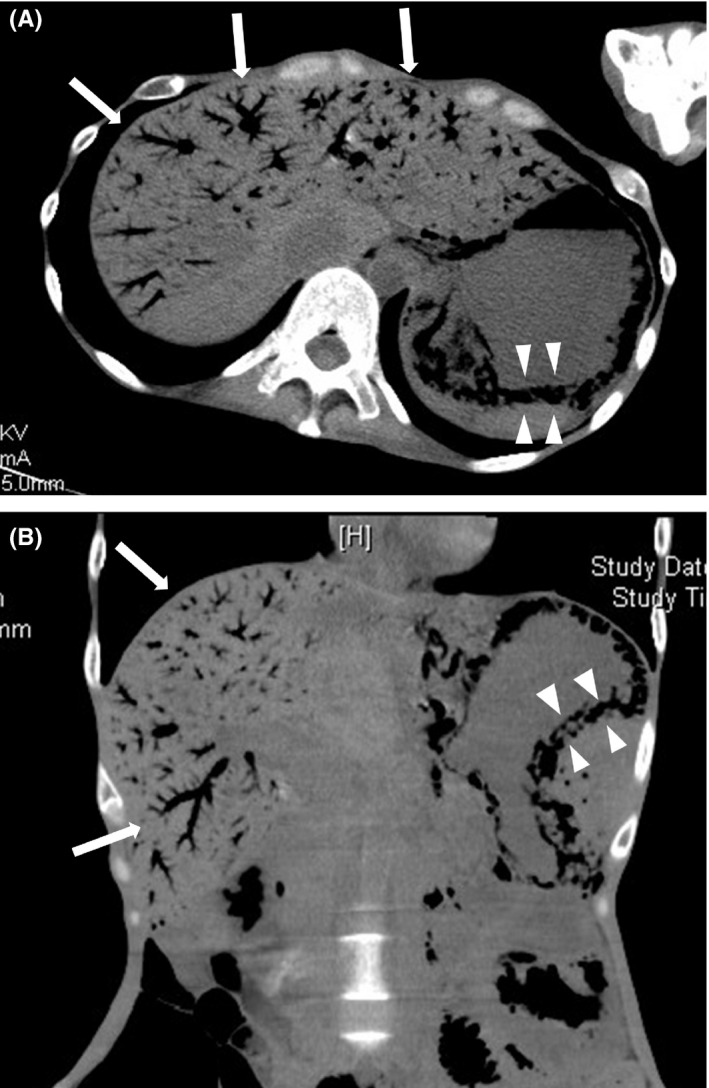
Abdominal computed tomography demonstrates gas within the gastric wall (white arrow heads) and the intrahepatic portal veins (white arrows). A, Axial view, B, Coronal view
